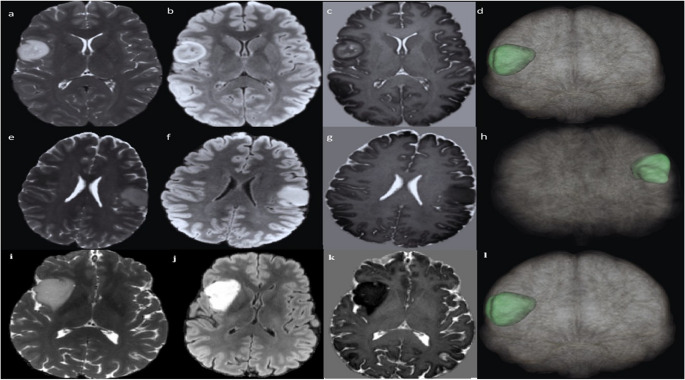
Representative cases demonstrating T2-FLAIR digital subtraction imaging and three-dimensional tumor volume rendering in non-enhancing low-grade glioma. Top row (a–d): IDH-mutant, 1p/19q non-codeleted, WHO Grade 2 diffuse astrocytoma in the right cerebral hemisphere (T2-FLAIR mismatch sign positive; radiomics model prediction: positive [astrocytoma]; neuroradiologist assessment: positive). a T2-weighted image showing homogeneous hyperintensity throughout the tumor, b FLAIR image revealing relative signal suppression of the central tumor with a hyperintense peripheral rim consistent with the T2-FLAIR mismatch sign, c T2-FLAIR digital subtraction image accentuating the signal intensity discrepancy between T2-weighted and FLAIR sequences within the tumor, d three-dimensional volume rendering depicting the segmented tumor volume (green overlay). Middle row (e–h): IDH-mutant, 1p/19q co-deleted, WHO Grade 2 oligodendroglioma in the left cerebral hemisphere (T2-FLAIR mismatch sign negative; radiomics model prediction: negative [non-astrocytoma]; neuroradiologist assessment: negative). e T2-weighted image, f FLAIR image demonstrating persistent hyperintensity without central signal suppression, g T2-FLAIR digital subtraction image showing minimal signal intensity difference between sequences, h three-dimensional volume rendering depicting the segmented tumor volume (green overlay). Bottom row (i–l): IDH-mutant, 1p/19q non-codeleted, WHO Grade 2 diffuse astrocytoma (T2-FLAIR mismatch sign positive; radiomics model prediction: positive [astrocytoma]; neuroradiologist assessment: negative). This discordant case demonstrates a tumor where the radiomics model correctly identified the astrocytoma molecular subtype, while the neuroradiologist visual assessment did not identify the T2-FLAIR mismatch sign due to incomplete signal suppression on FLAIR. i T2-weighted image showing tumor hyperintensity, j FLAIR image demonstrating partial signal suppression that did not meet strict visual mismatch criteria, k T2-FLAIR digital subtraction image revealing quantifiable signal intensity differences captured by the radiomics model, l three-dimensional volume rendering depicting the segmented tumor volume (green overlay)
